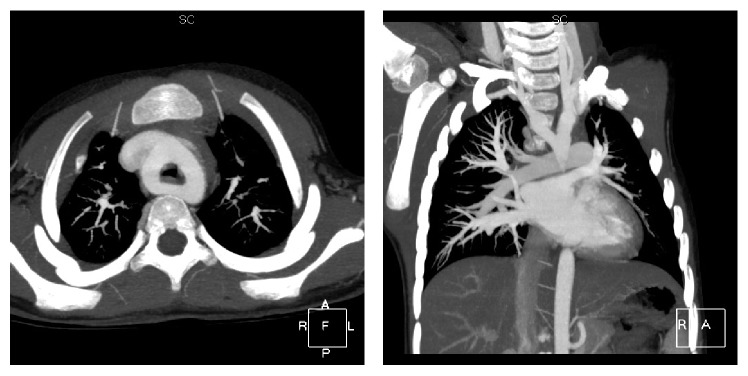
Contrast-enhanced CT showing the double aortic arch.
